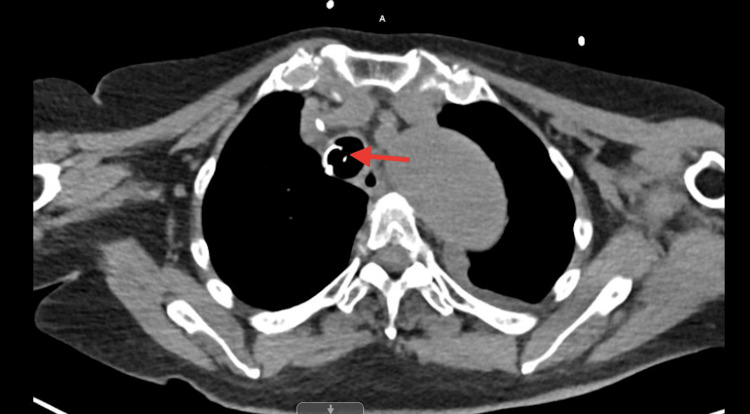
A CT angiogram of the abdomen and thorax revealing a stable type B aortic dissection
